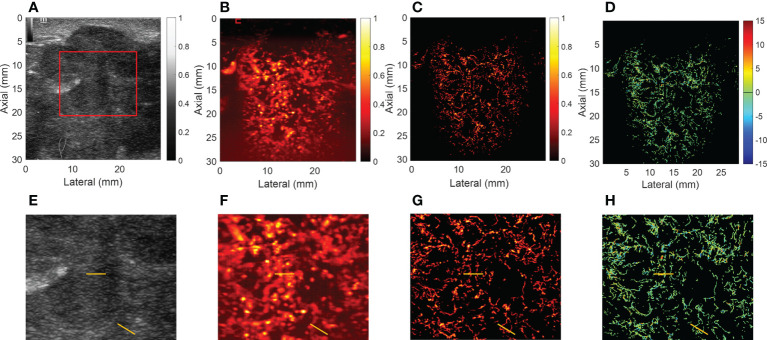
Ultrasound images of a representative malignant breast mass. (A) B-mode image. (B) Contrast-enhanced ultrasound image. (C) Super-resolution image. (D) Super-resolved velocity image. (E–H) shows the zoomed-in regions of (A–D) as the red box indicated in (A). Yellow lines in (E–H) highlight the resolution improvement among the images.
